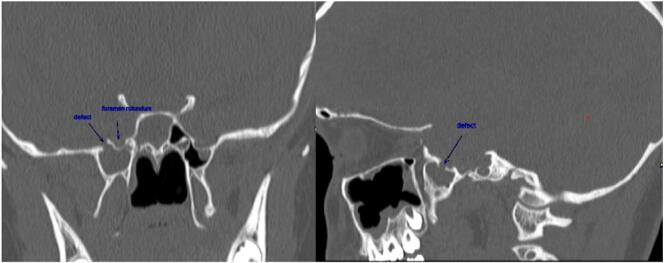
CT scan coronal and sagittal view showing meningoencephalocele through a defect in the roof of the right LRS sinus.
